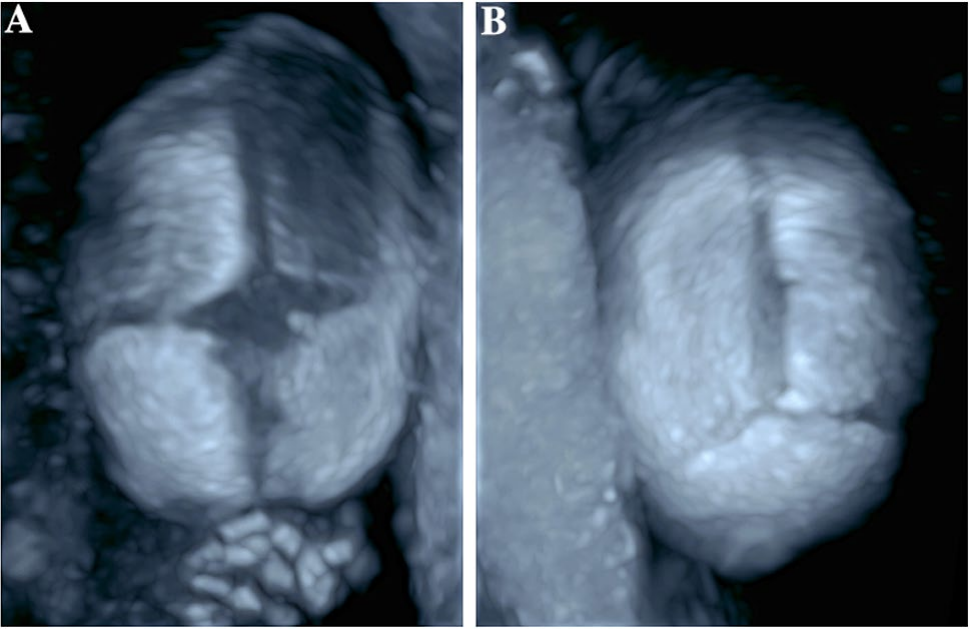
Normal findings of a fetal skull imaged by three-dimensional ultrasonic skeletal imaging mode. Image of the anterior fontanelle with adjacent sutures—sutura frontalis, sutura sagittalis and sutura coronalis (a). Image of the posterior fontanelle with adjacent sutures—sutura sagittalis, sutura lambdoidea (b)
